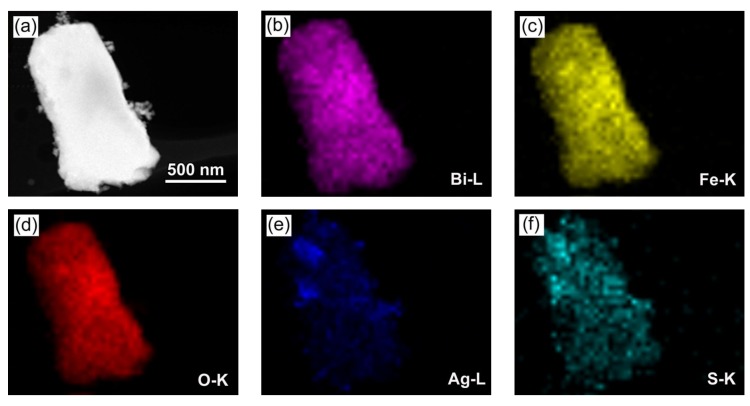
(a) Dark-field scanning TEM (DF-STEM) image of 15% Ag_2_S/BiFeO_3_. (b–f) The energy-dispersive X-ray elemental mapping images of the region shown in (a).
